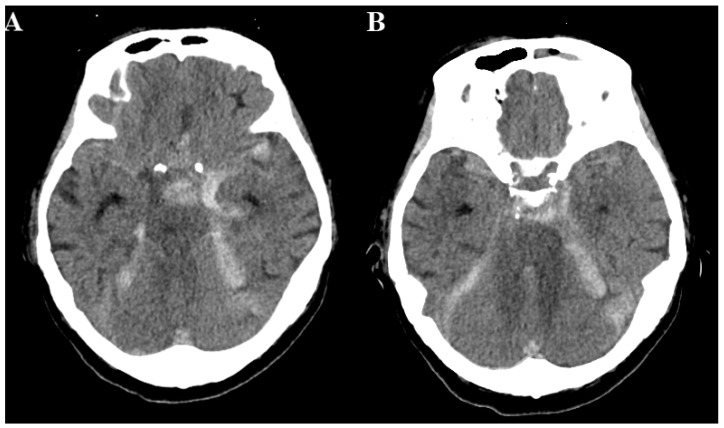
(A,B) Cranial computed tomography with contrast enhancement. Diffuse subarachnoid hemorrhage was noted.
